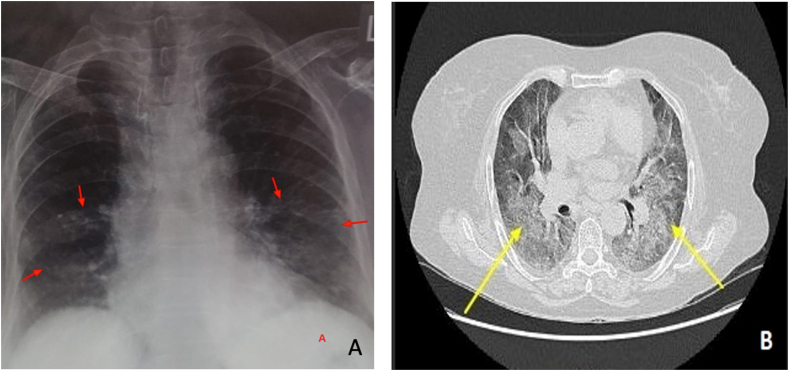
(A). X ray of a post-COVID-19 patient. Red arrows indicate fibrotic changes, more prominent in the basal regions of both lungs. (B). High resolution CT-scan suggest massive fibrosis, diffuse symmetrical ground glass opacities (yellow arrows) involving both lung fields along with septal thickening. (For interpretation of the references to colour in this figure legend, the reader is referred to the Web version of this article.)
